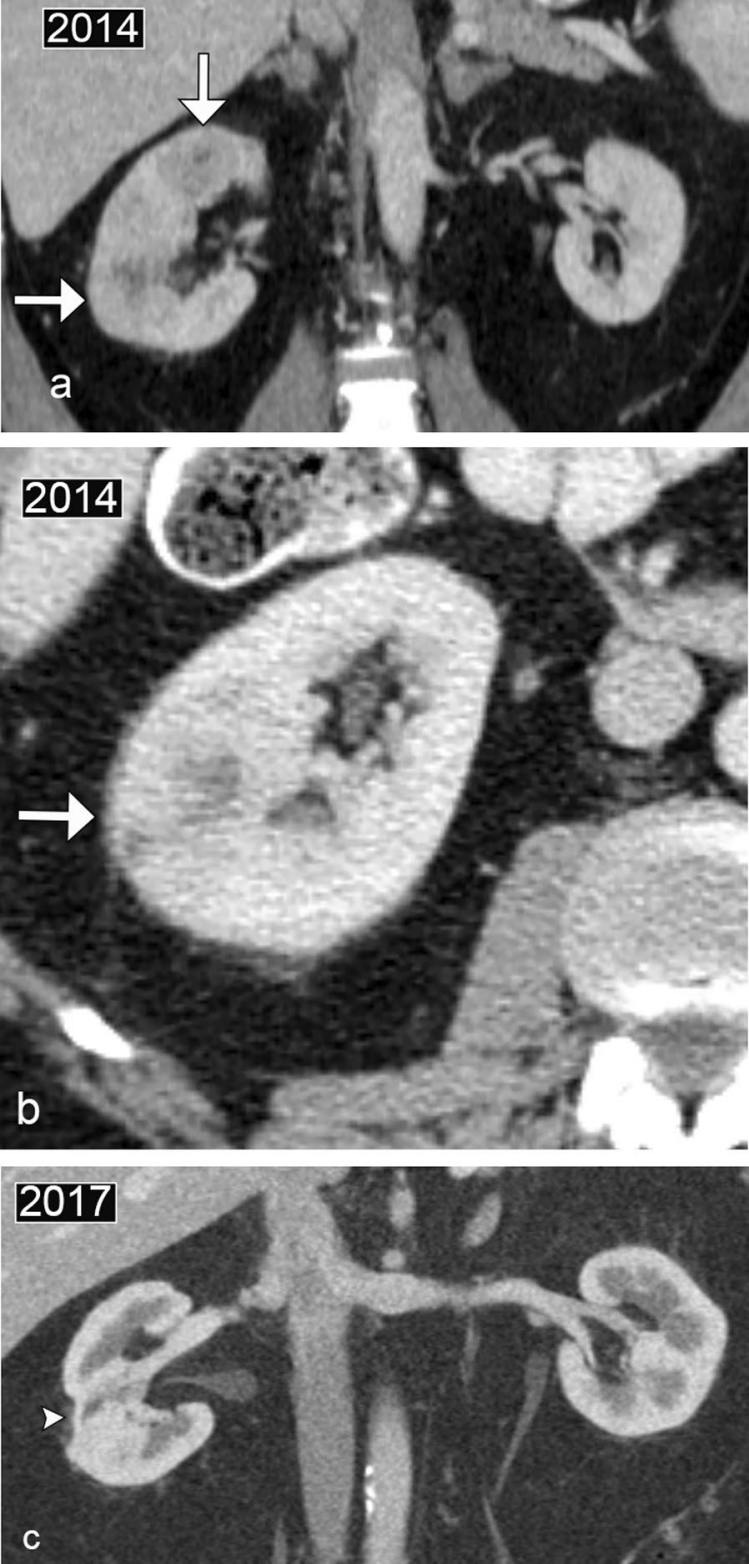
Pyelonephritis and renal abscesses.* Coronal (a) and axial (b) contrast CT images in 2014 of the abdomen demonstrate a delayed right nephrogram with cortical heterogeneity. Suggestion of mass-like areas in the right kidney with perinephric stranding (arrows) identified, mimicking the multiple masses of renal lymphoma. This was presumed to be related to pyelonephritis with renal abscesses based on clinical presentation. Follow-up coronal contrast CT in 2017 (c) demonstrates focal parenchymal scarring in the region of the right lower pole mass as a result of prior infection (arrowhead)
